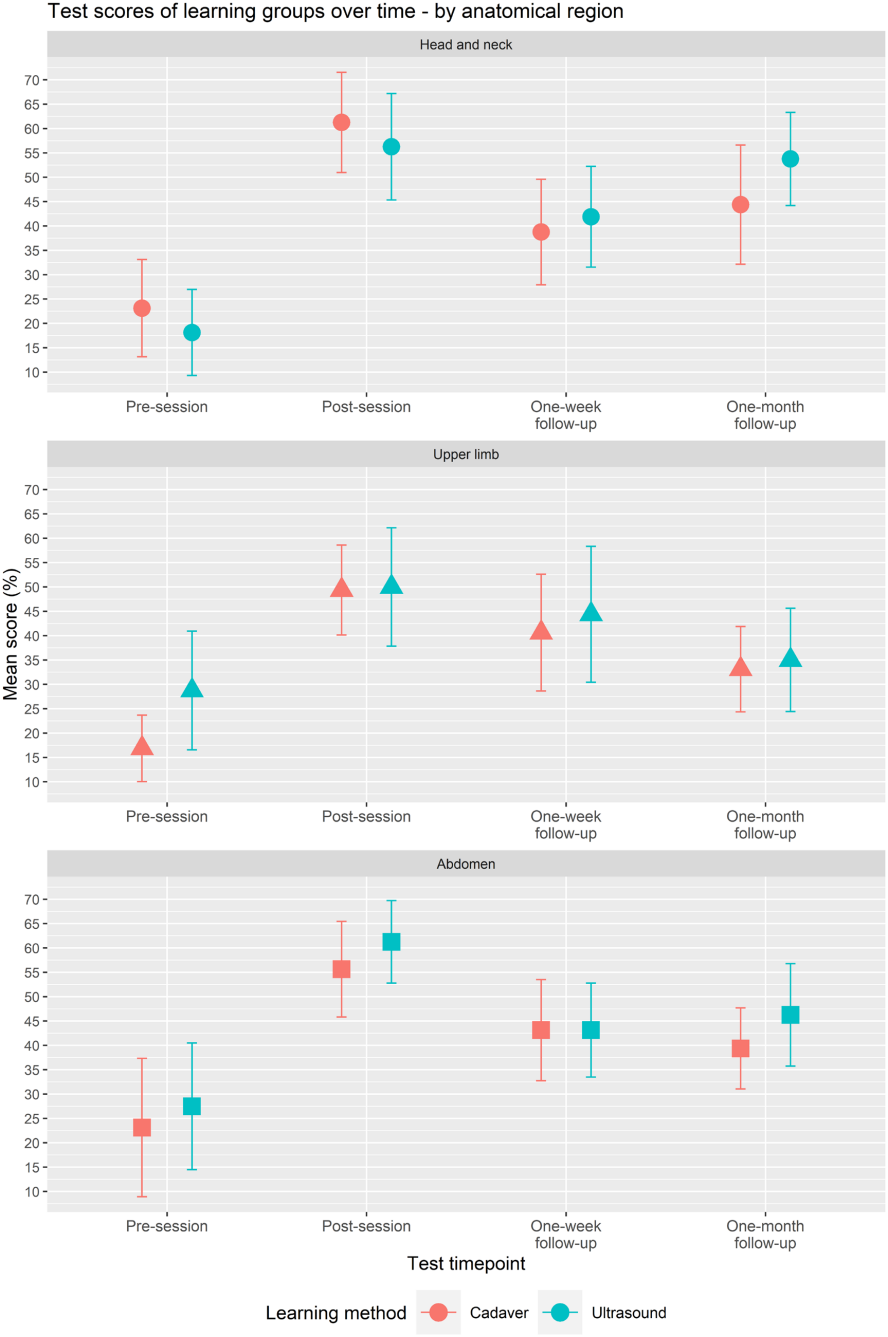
Mean knowledge test scores for specific anatomical regions (head and neck, upper limb, and abdomen) at each time point throughout the study are shown in red for the cadaver‐based learning group, and blue for the ultrasound‐based learning group. Whiskers represent the 95% confidence intervals for each of the scores.
